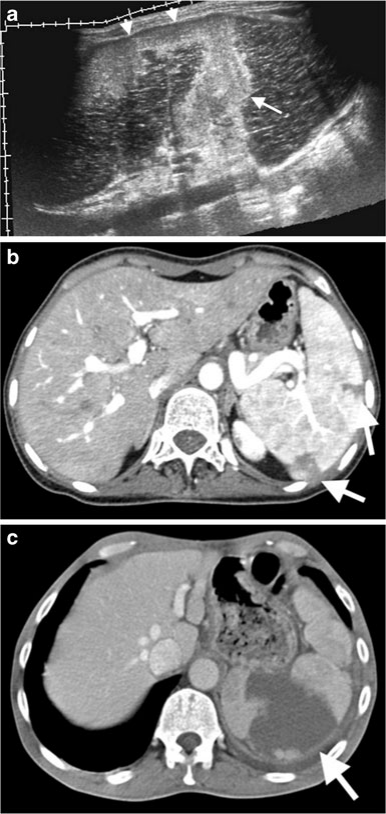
Splenic infarcts with various clinical conditions. (a) Panoramic view of gray scale US of a 54-year-old man with atrial fibrillation demonstrates hyperechoic infarct (*arrow*) traversing splenic parenchyma from the hilum to a peripheral part of the spleen. Neighbouring subcapsular portion of the spleen exhibits a hyperechoic appearance (*arrowheads*) representing subcapsular infarct. (b) Axial contrast-enhanced CT of a 21-year-old woman with polyarteritis nodosa reveals peripheral wedge-shaped low attenuated infarcts (*arrows*). (c) Axial contrast-enhanced CT image of a 79-year-old man with atrial fibrillation reveals a peripheral wedge-shaped low attenuated infarct (*arrow*)
